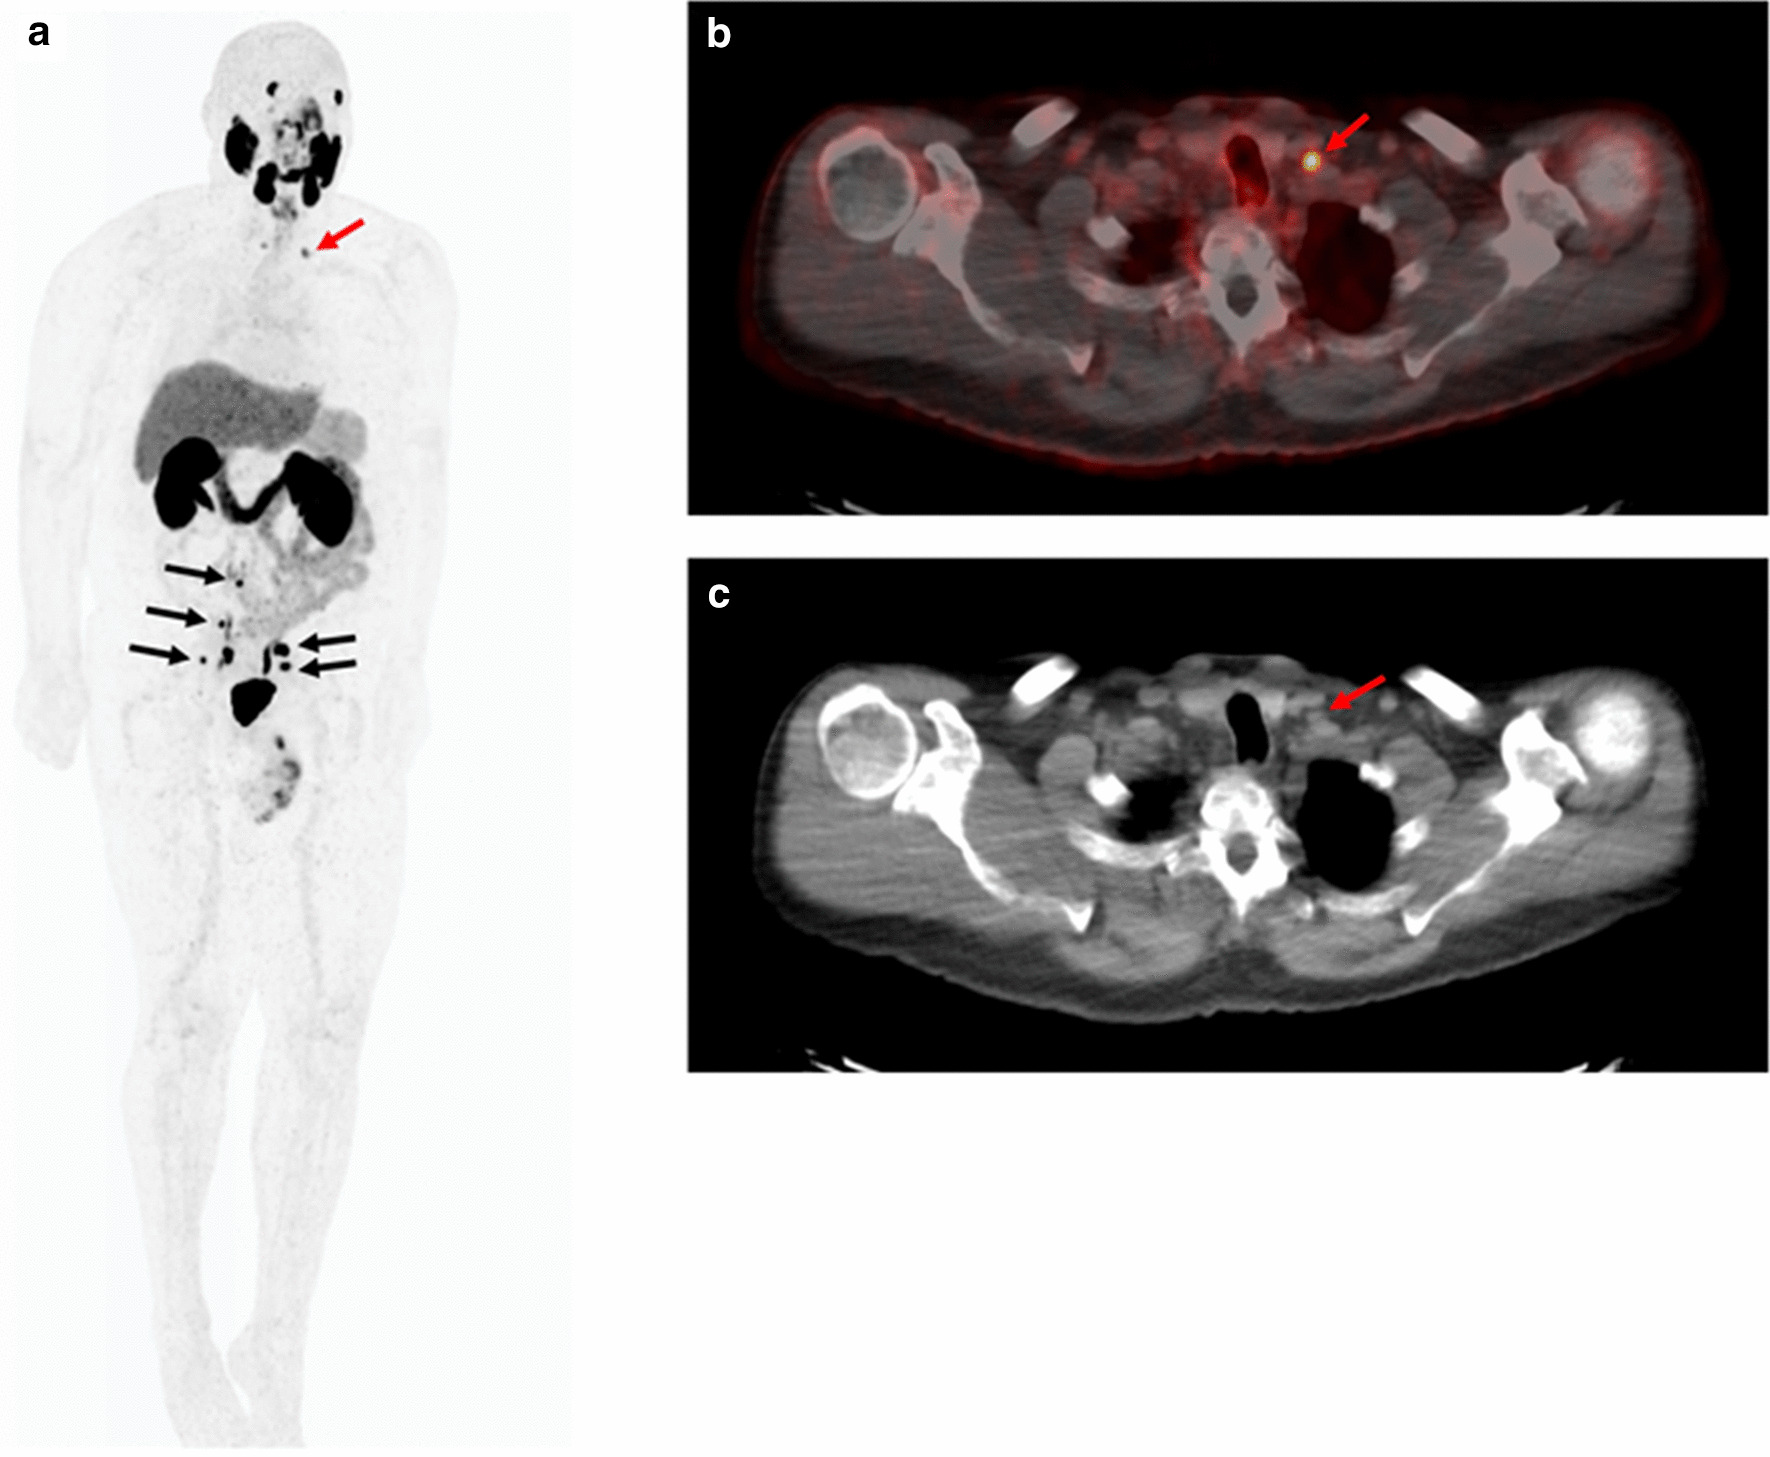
66-year-old male with serum PSA of 4.83 ng/ml after radical prostatectomy and salvage radiation therapy. ^18^F-DCFPYL PET/CT shows focal radiotracer uptake within bilateral pelvic lymph nodes (black arrows) (a) and in a normal sized supraclavicular lymph node (red arrows) (a–c)
